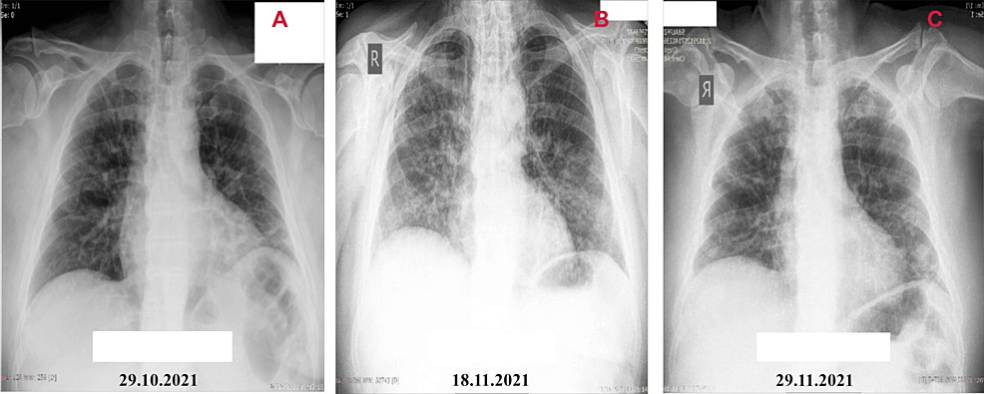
Radiography of lungs and heart conducted during the stay in the hospital А) Bilateral diffuse interstitial changes; В) Bilateral interstitial changes are more pronounced, affecting almost all lung fields; С) Bilateral interstitial changes are reduced compared to В.
